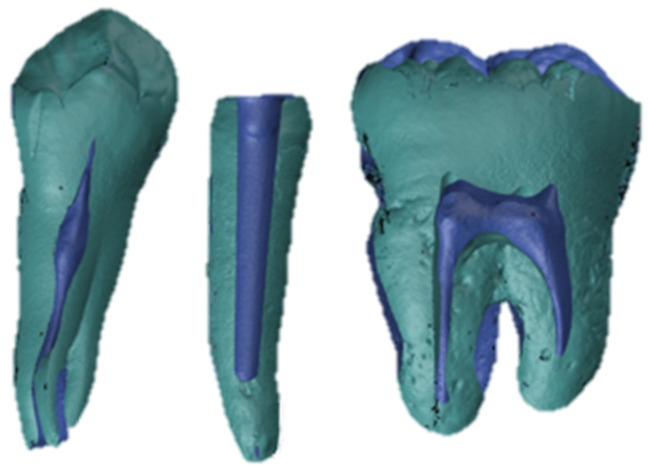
µ-CT reference dataset of a test model with prepared study tooth and adjacent teeth in representative cross section.
